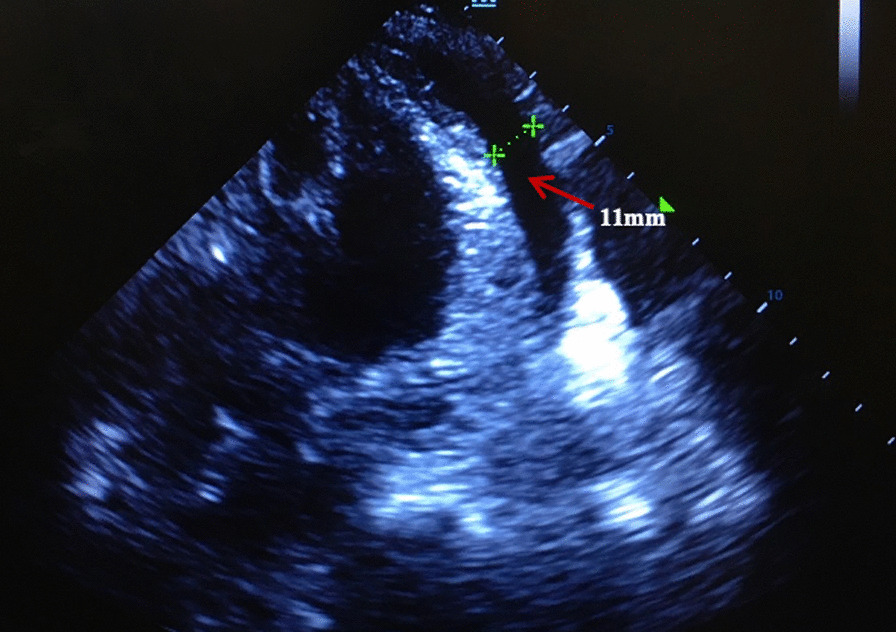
The echocardiogram showing pericardial effusion (red arrow) during diastolic phase mainly distribution around the posterior wall of left ventricle
